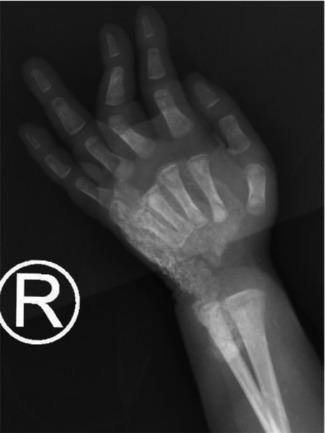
X-ray showing the dorsum of the Right hand.
